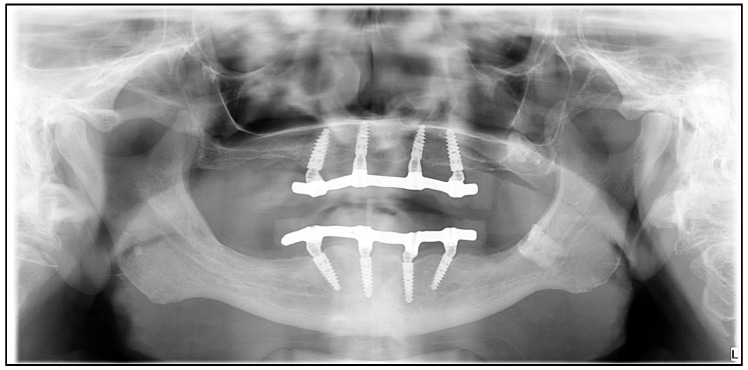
Panoramic radiograph of the patient 4 years post-implant placement.
